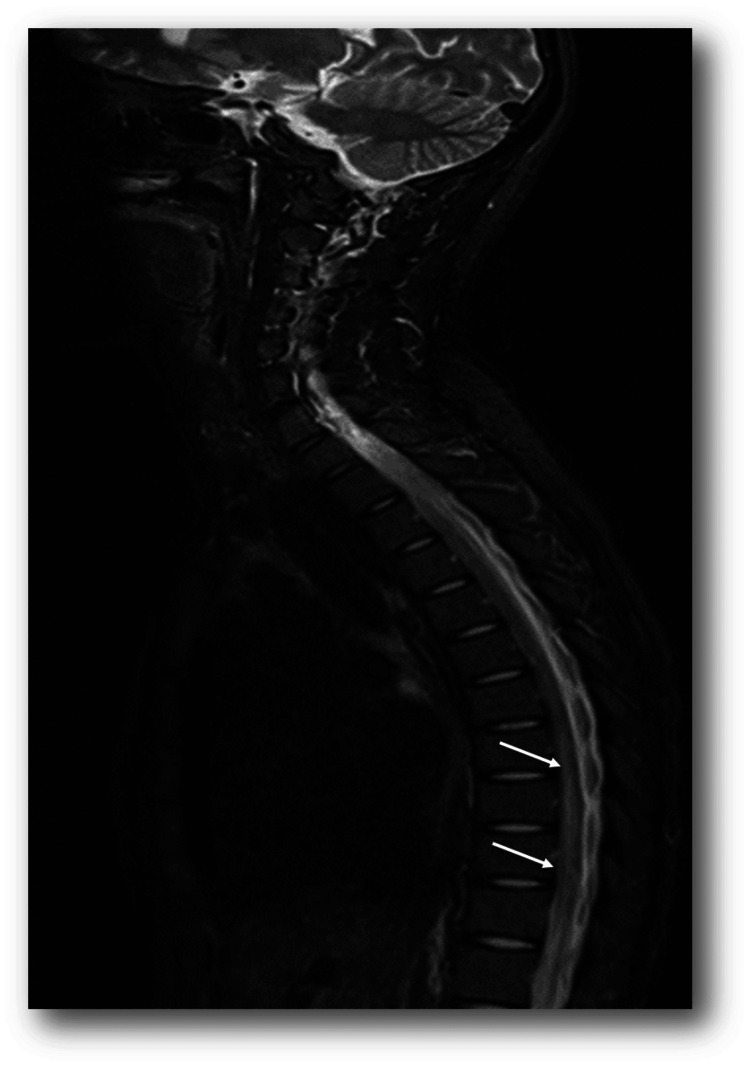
T2-weighted sagittal image revealing an extensive area with a slight increase in diameter that presents high signal intensity from the T6 to T10 level (white arrows).
